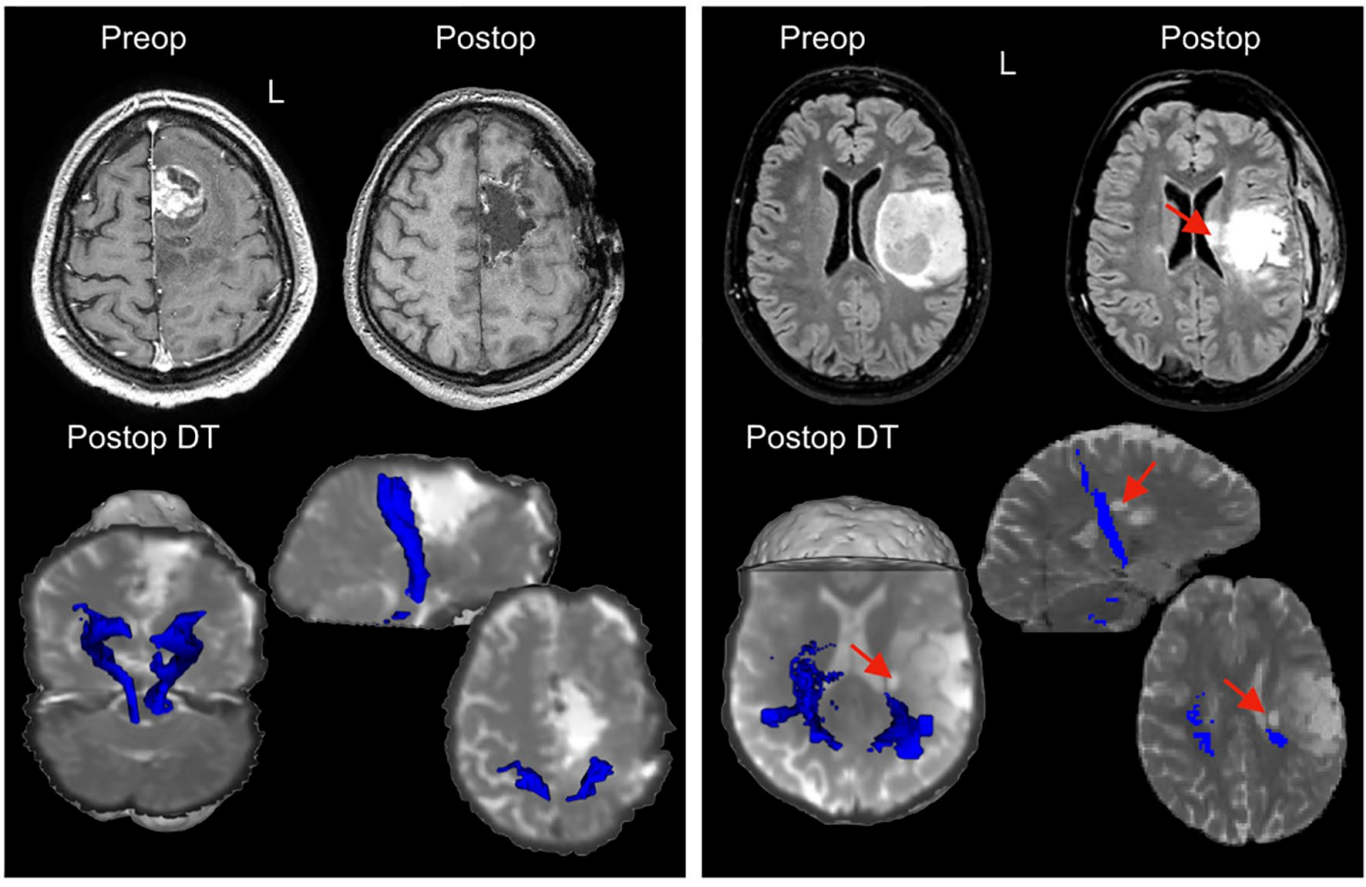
Post-operative diffusion tractography to inform recovery potential in two patients with post-operative deficits. Left panel: A 42-year-old right handed male developed speech and motor deficits during resection of a radiologically transforming glioma in the medial frontal lobe. Speech recovered quickly over the first 3 days following surgery. Right hand function was absent immediately following surgery (0/5, MRC scale) and right leg function was significantly impaired (2/5). Post-operative diffusion tractography (DT) acquired 24 h after surgery showed preserved corticospinal (CST) fibers, supporting the diagnosis of a supplementary motor syndrome likely to recover. Hand function gradually returned on the 6th post-operative day; leg strength returned to normal by 1 month. Right panel: A 34-year-old right handed female developed sudden limb weakness. Immediate post-operative MRI identified an area of infarct affecting the corona radiata and body of the caudate nucleus. Post-operative DT demonstrated an asymmetrical CST, with fewer detected tracts adjacent to the infarct. Residual connections reaching the primary motor cortex, however, suggested some recovery potential. Hand function improved over the first 10 days after surgery, with additional gains in lower limb function with therapy which continued for 3 months post-surgery. However, she was still occasionally using a stick to walk
